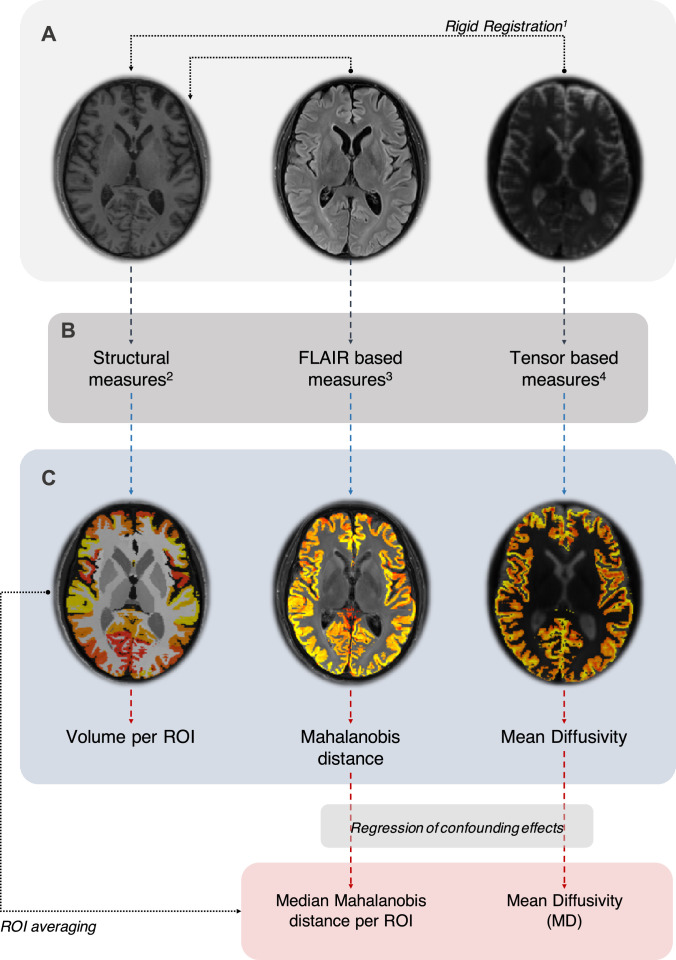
Representation of the framework adopted for feature extraction. A: data preprocessing step, including rigid registration using (1) *NiftyReg* Modat et al. (2014). B: Feature extraction per MRI sequence, applying (2) GIF algorithm (Cardoso et al., 2011) to T1, using (3) BaMoS algorithm to extract the intensity distributions of FLAIR (Sudre et al., 2015), and computing the diffusion tensor from DWI. C: Quantitative features computed from the images obtained in the section B of the framework.
